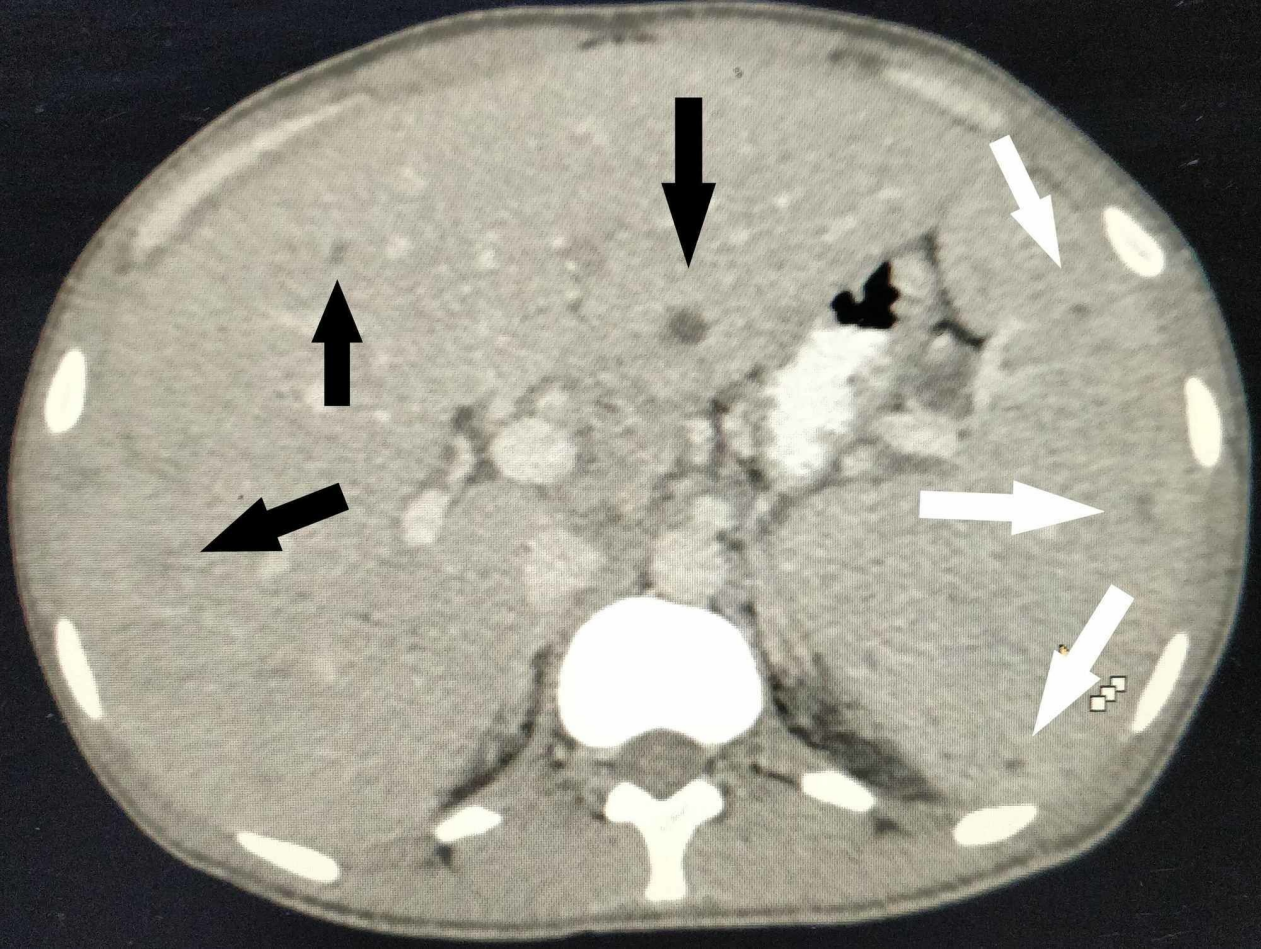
Axial section of contrast-enhanced CT scan of the abdomen Microabscesses in the liver (black arrows) and spleen (white arrows) visible as small round, hypodense lesions with minimal peripheral enhancement.
